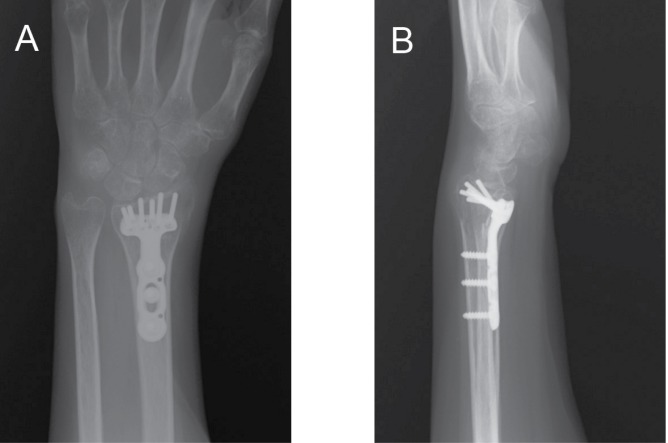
Standardised three-month postoperative posteroanterior (A) and lateral (B) radiography of a fractured distal radius that has been stabilised with a DVR^®^ plate
